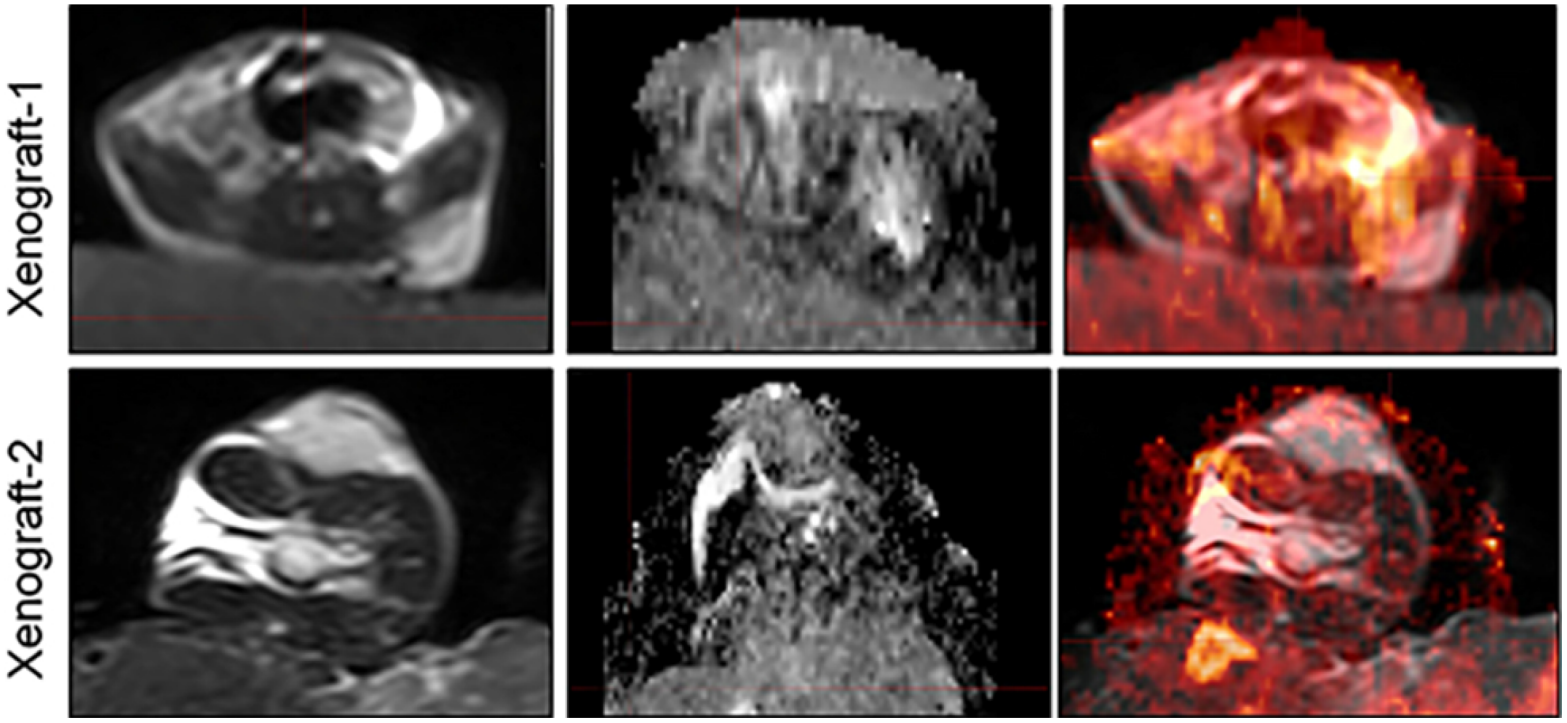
Representative images of MRI and ADC, as well as the deformed images using DIR.
